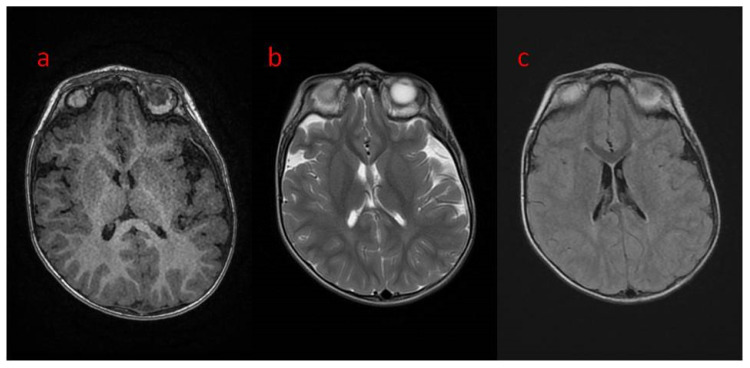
(a–c) Brain MRI in transverse planes: (a) normal in T1; (b,c) unfished myelinisation in peritrigonal white matter in (b) T2; (c) T2 FLAIR.
